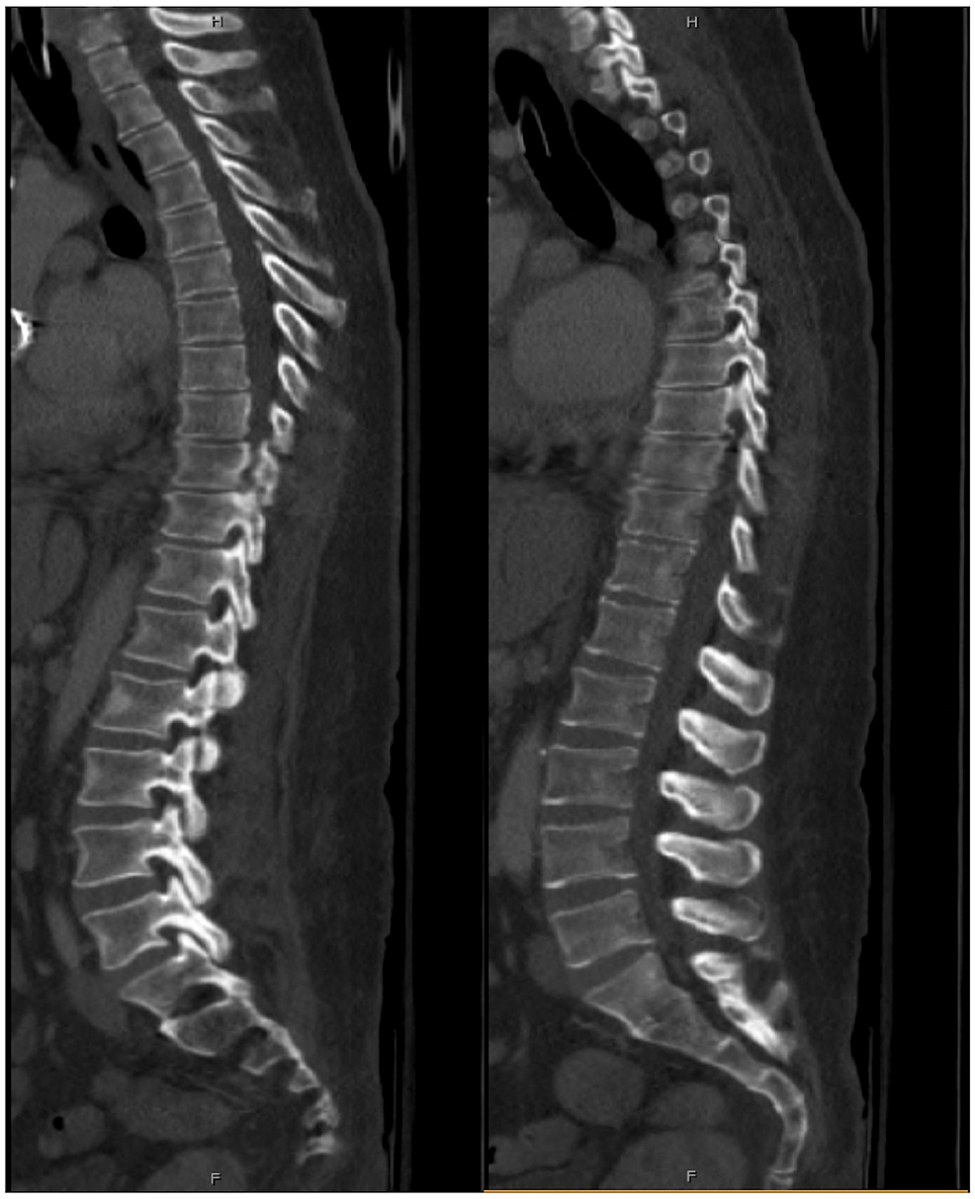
Initial Computed Tomography Scan of the Thoracic Spine Multiple sagittal views of the pre-operative computed tomography scan of the thoracic spine were found to be completely unremarkable.
